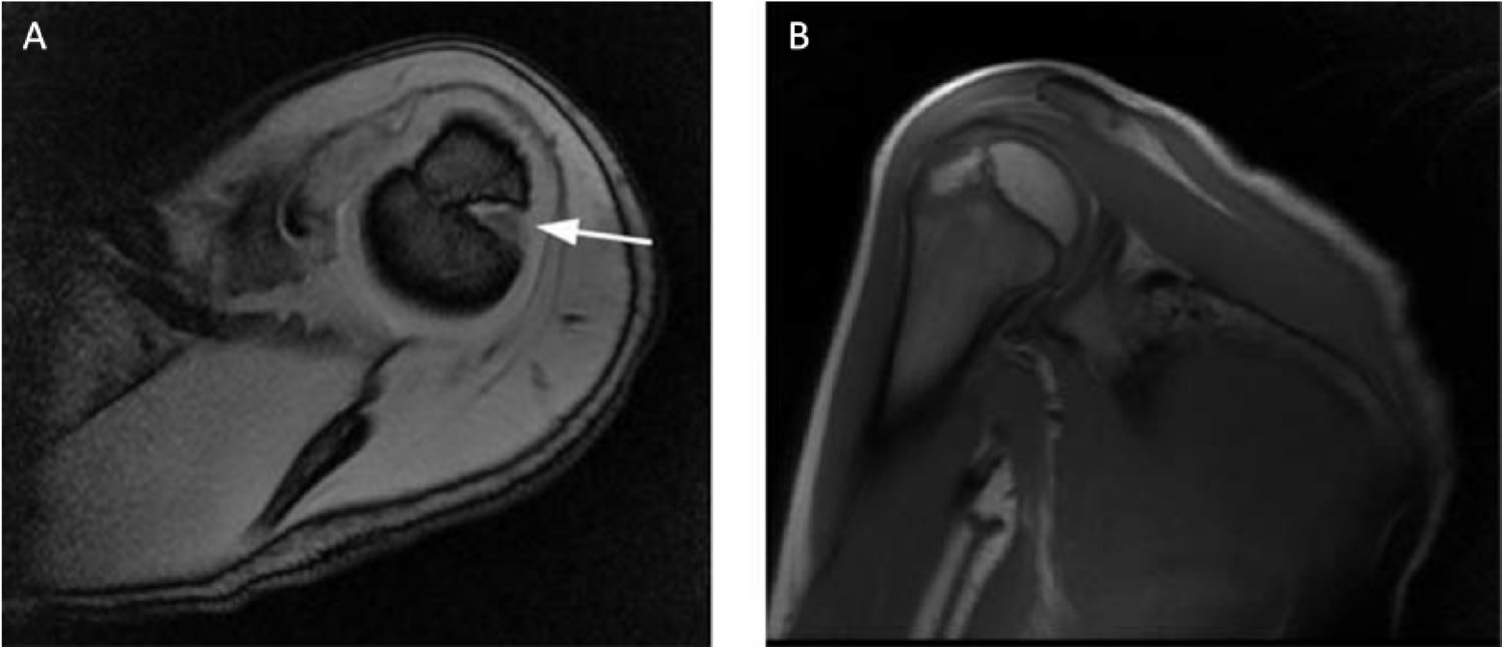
Axial (A) and coronal (B) T1-weighted MRI images of the shoulder in a 9-year-old patient. The images were obtained one week after reduction of an anterior shoulder dislocation was performed in the emergency department. The axial view demonstrates a Hill-Sachs lesion at the proximal humeral physis (arrow). This lesion is not visible on the coronal view. Figure taken from Li et al. 2013 [36]. Reprinted with permission from Wolters Kluwer Health, Inc [36]
